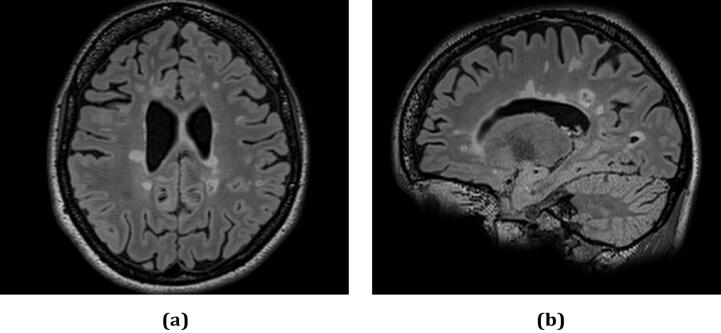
MS lesions in brain MRI axial (a) and sagittal (b) view. The figure comes from a dataset described in Lesjak et al. (2016).
